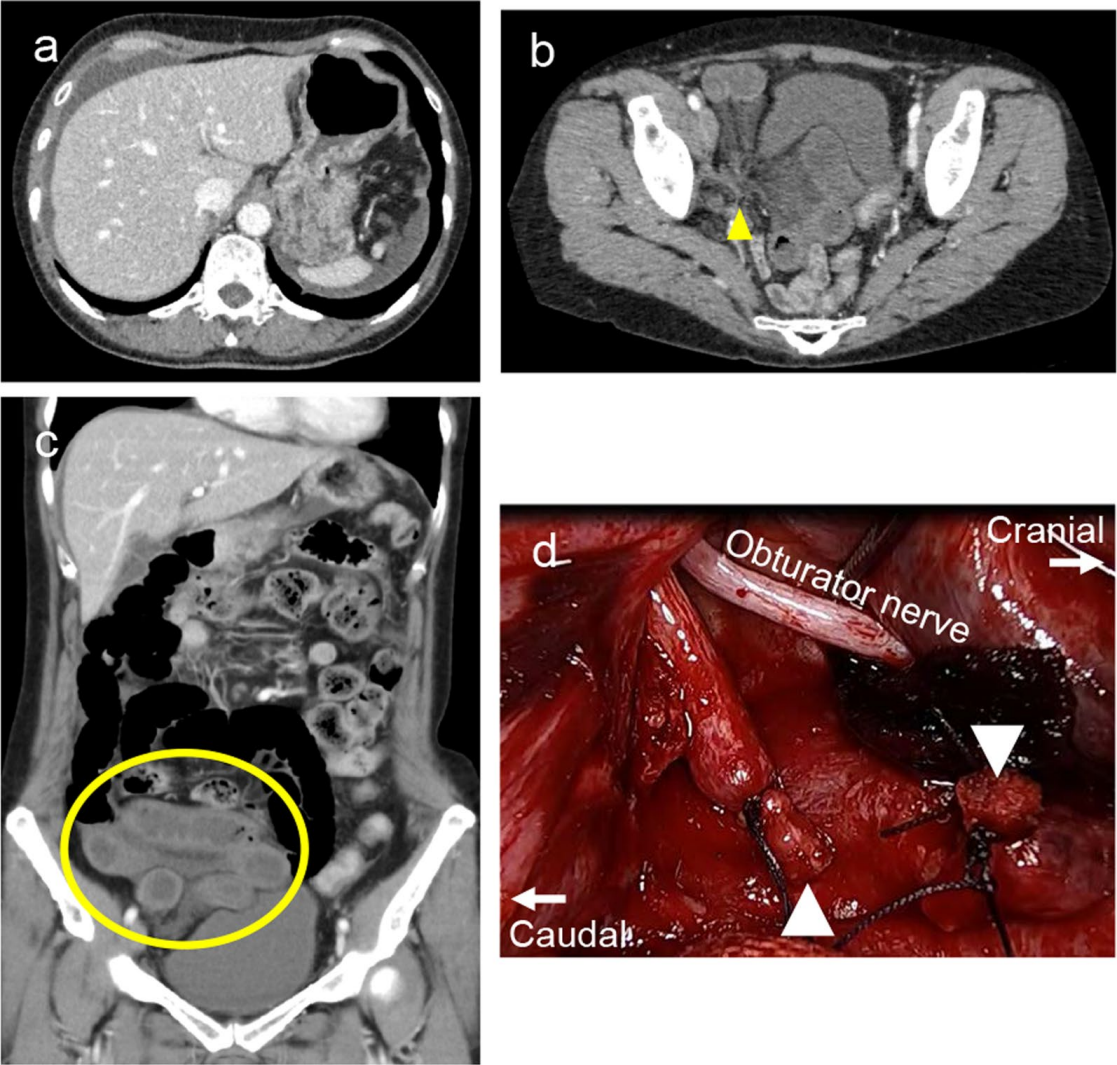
Abdominal CT scan images before urgent surgery (a–c) and a laparoscopic view after resection of umbilical artery (d) of the case 2 patient. a Massive ascites are found on the liver surface. b and c Axial (b) and coronal view (c) of the strangulated small bowel. The yellow arrowhead and circle denote the strangulated origin and edematous mesentery and intestine. d Umbilical artery stump (white arrowhead) after resection of the incarcerated small bowel and a cord
